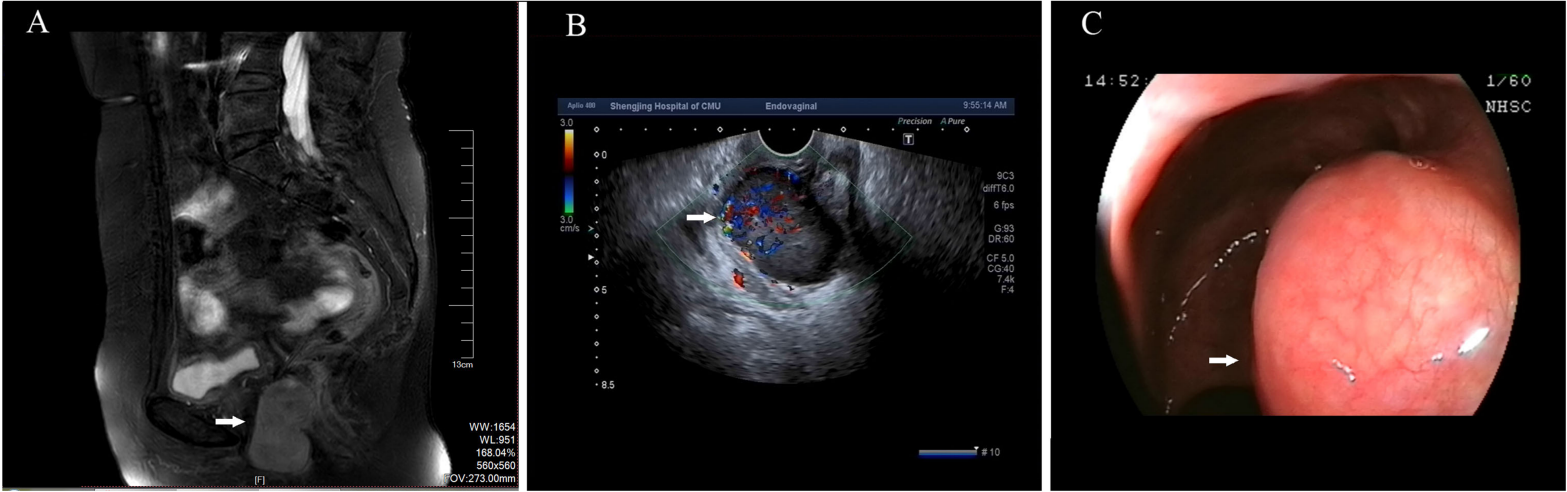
Imaging examinations show the location of the rectal GISTs. a The results of pelvic MR imaging reveal that the tumor (arrow) was located in the vaginal-rectal space and protruded from the anterior rectal wall. b The results of transvaginal ultrasound show that the tumor (arrow) protruded from the posterior of the lower vaginal wall. c Colonoscopy results show that the tumor originated from the rectal dentate line
